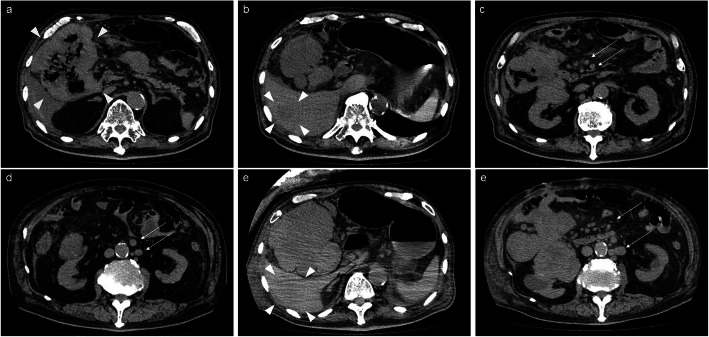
Computed tomography scan. Computed tomography shows a large intra-abdominal mass infiltrating into the colon and retroperitoneum (a, arrowhead) as well as the low-density area of the liver (b, arrowhead), peritoneal nodule (c, arrow), and lymphadenopathy in several paraaortic lymph nodes, suggesting metastases or dissemination (d, arrow). Pneumoperitoneum was visible, suggesting colon perforation near the tumor. Liver tumor, metastatic lymph node, and peritoneal dissemination were enlarged after 1 month (e, f, arrow, arrowhead)
